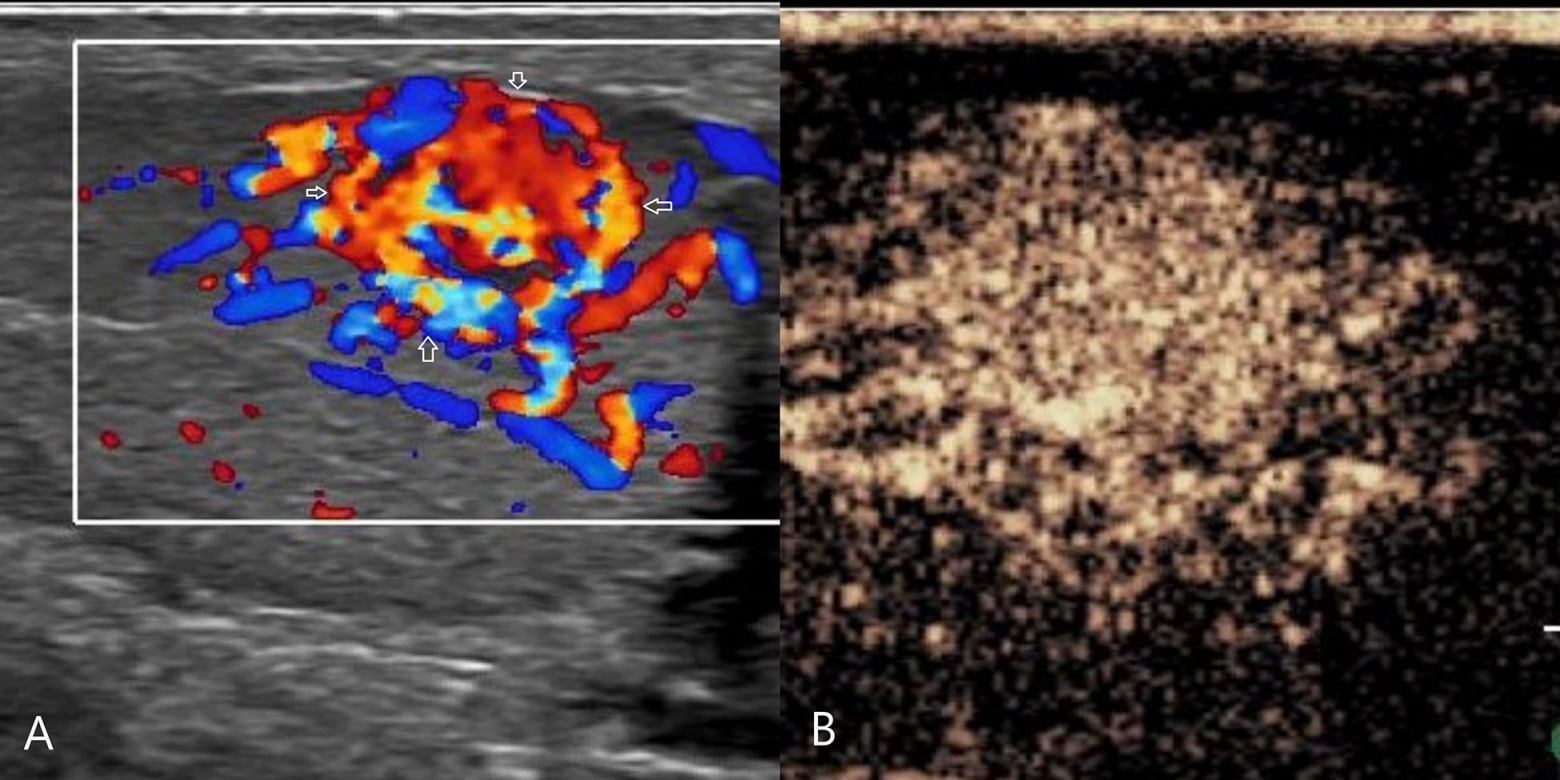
Leydig cell tumor. (A) CDFI: hypoechoic mass in the left testis, abundant blood flow, with annular blood flow around(arrow). (B) CEUS: uniform and high enhancement, and no obvious necrotic area was found. CDFI, Color Doppler Flow Imaging; CEUS, contrast-enhanced ultrasonography.
